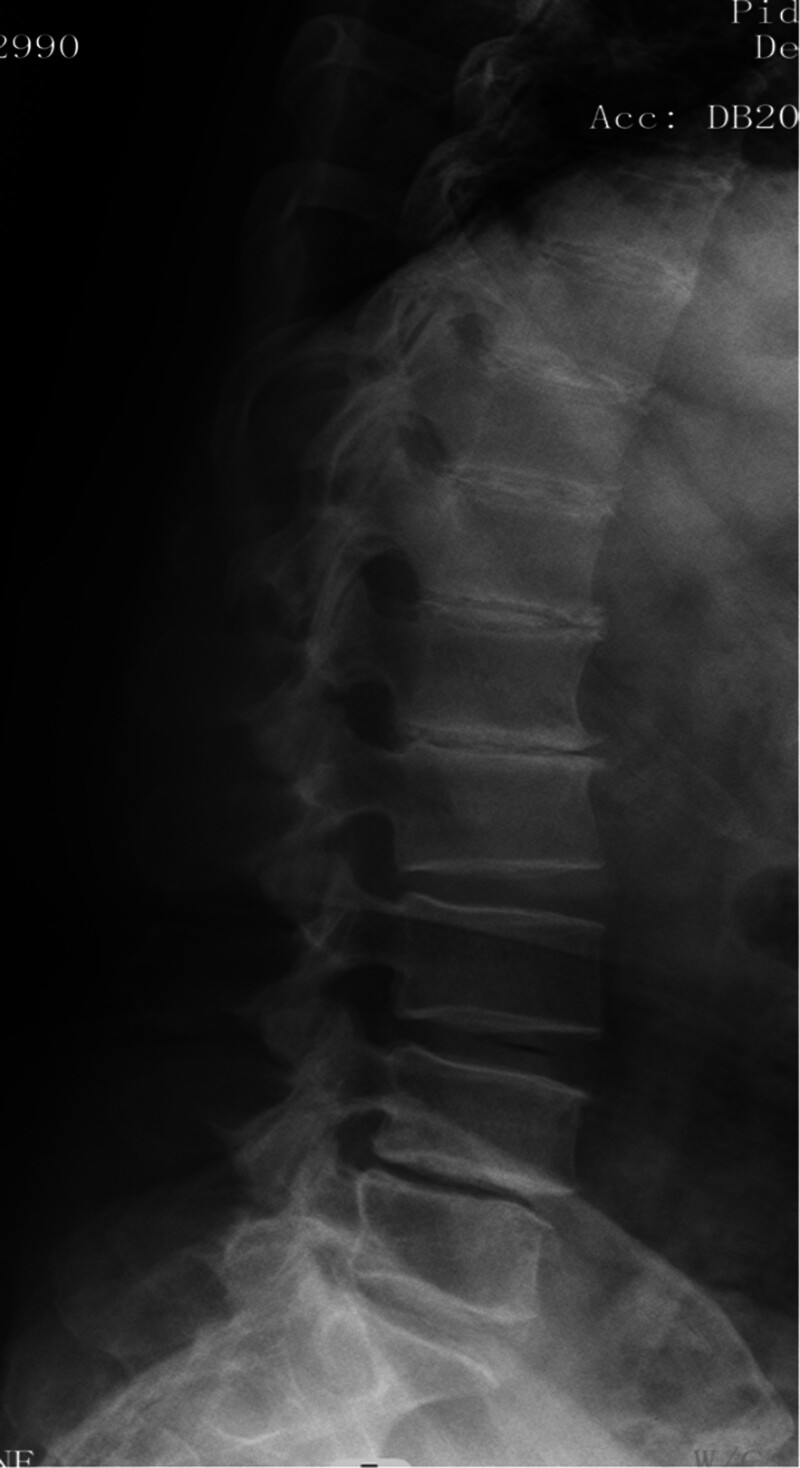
X-ray plain film showed intra-discal calcification or vacuum in multiple discs along with end plate sclerosis.
